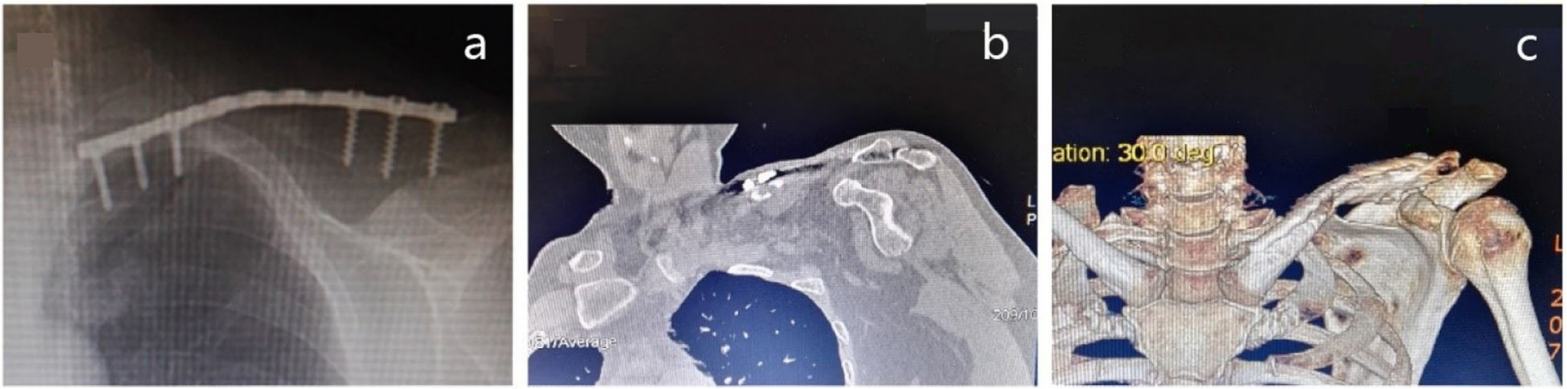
a Clavicle nonunion with plate fixation at 9 months. b Nonunion confirmed by CT scan. c Nonunion was showed in the 3D reconstruction of the clavicle
